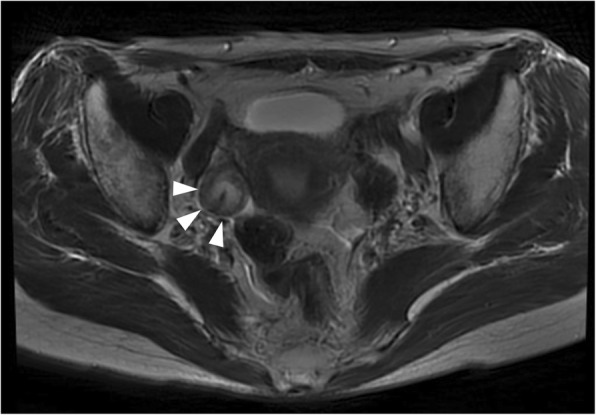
Magnetic resonance imaging revealed a right ovarian mass 25 mm in diameter with a solid component (arrowhead)
